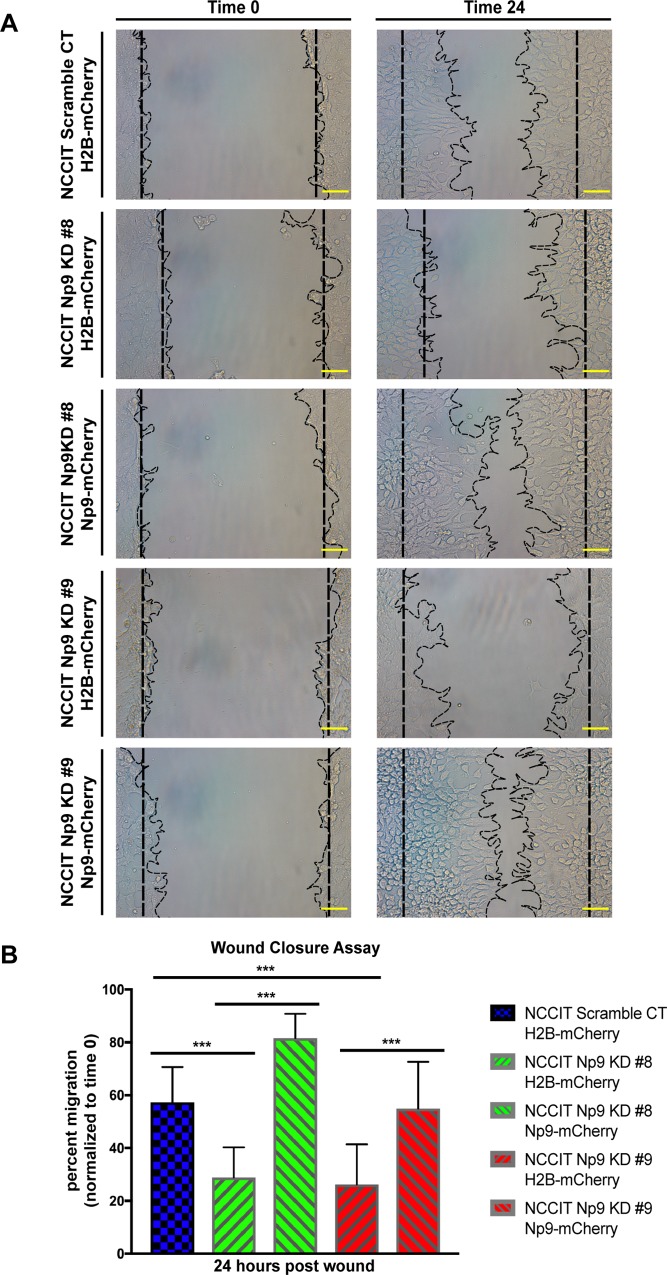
Re-introduction of Np9 into teratocarcinoma cells rescues the migration phenotype. (A) NCCIT scramble, Np9 KD clones 8 and 9 with H2B-mCherry control clones and NCCIT Np9 KD clones 8 and 9 with Np9-mCherry clones were used for the migration assay. A total of 200,000 cells for each cell type were transferred into a 12-well plate. The following day, a scratch was created down the middle, and pictures were taken at time 0 and at 24 hours. The images are representative of 2 independent experiments done in duplicate. Scales bars, 100 μm. (B) The wound was measured at time 0 and at 24 hours. The percent of migration was plotted over time 0. The measurements are representative of 2 independent experiments done in duplicate. The width of the wound was measured for each sample: scramble CT-H2B-mCherry (n = 83), Np9 KD #8 H2B-mCherry (n = 135), Np9 KD #8 Np9-mCherry (n = 156), Np9 KD #9 H2B-mCherry (n = 160), and Np9 KD #9 Np9-mCherry (n = 145). The re-introduction of Np9 into Np9 KD cells rescued the migratory phenotype of the NCCIT cells almost to the level of the scramble control, and in the case of Np9 KD clone 8 complemented with Np9-mCherry, the re-introduction of Np9 resulted in faster migration compared to the scramble control. T bars denote the standard deviations of the means; P values were determined using student t-test analysis. P*<0.0001.
